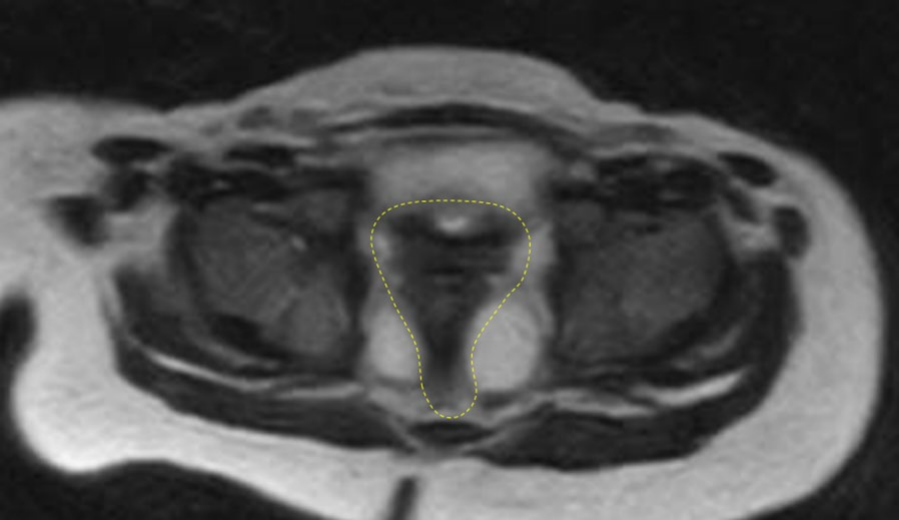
Preoperative MRI scans. T2-weighted images (axial view). Muscle complex are outlined by dot line
